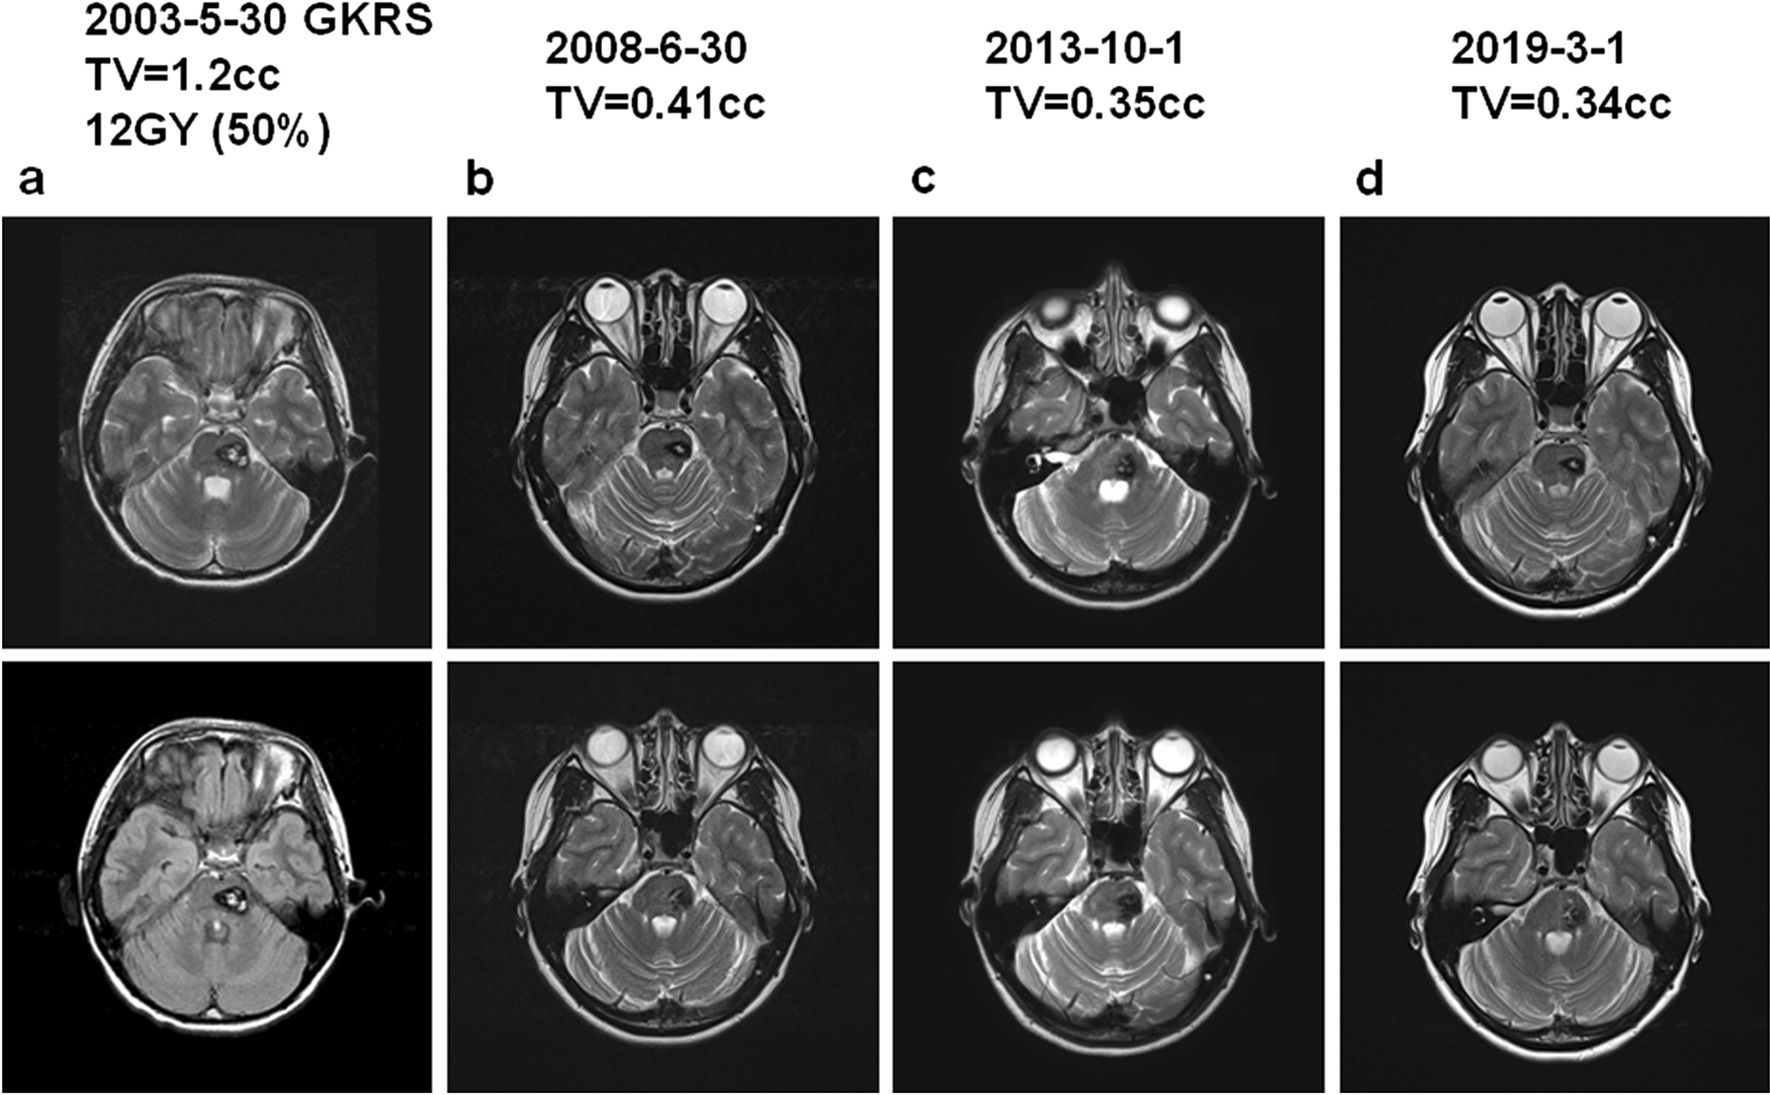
Cavernoma treated with GKRS with decreased size. A 13 year old girl suffered right side limb weakness with muscle power of grade IV and received GKRS with the regression of nidus. a MRI imaging of T2, Flair at the time of GKRS with radiation volume of 1.2 cc with 12 Gy in 50% line, b MRI imaging of T2 and Flair 6 years after GKRS with nidus volume of 0.41 cc, c MRI imaging of T2 and Flair 10 years after GKRS with nidus volume of 0.35 cc and d MRI imaging of T2 and Flair 16 years after GKRS with nidus volume of 0.34 cc
